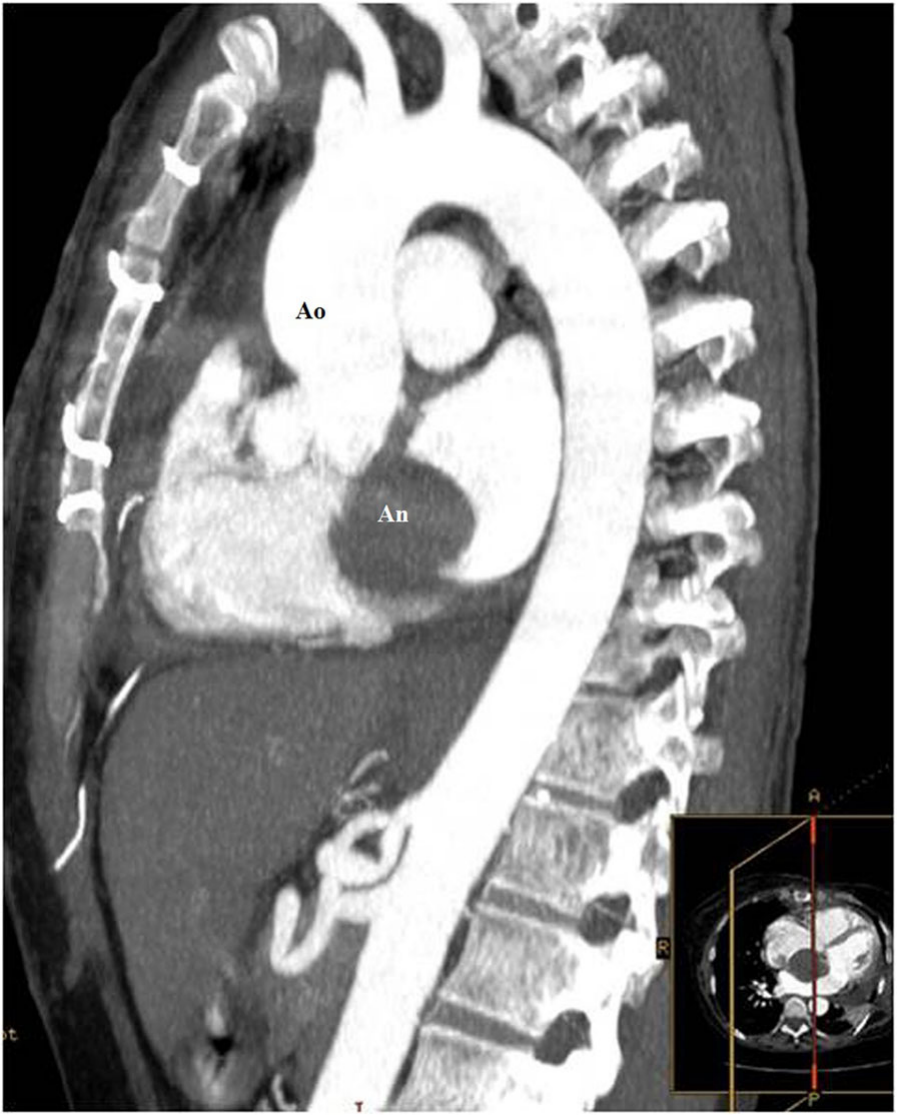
Postoperative contrast-enhanced angio-computed tomography demonstrating total obliteration of the aneurysmatic sac. *An* aneurysm of the coronary sinus, *Ao* aorta
